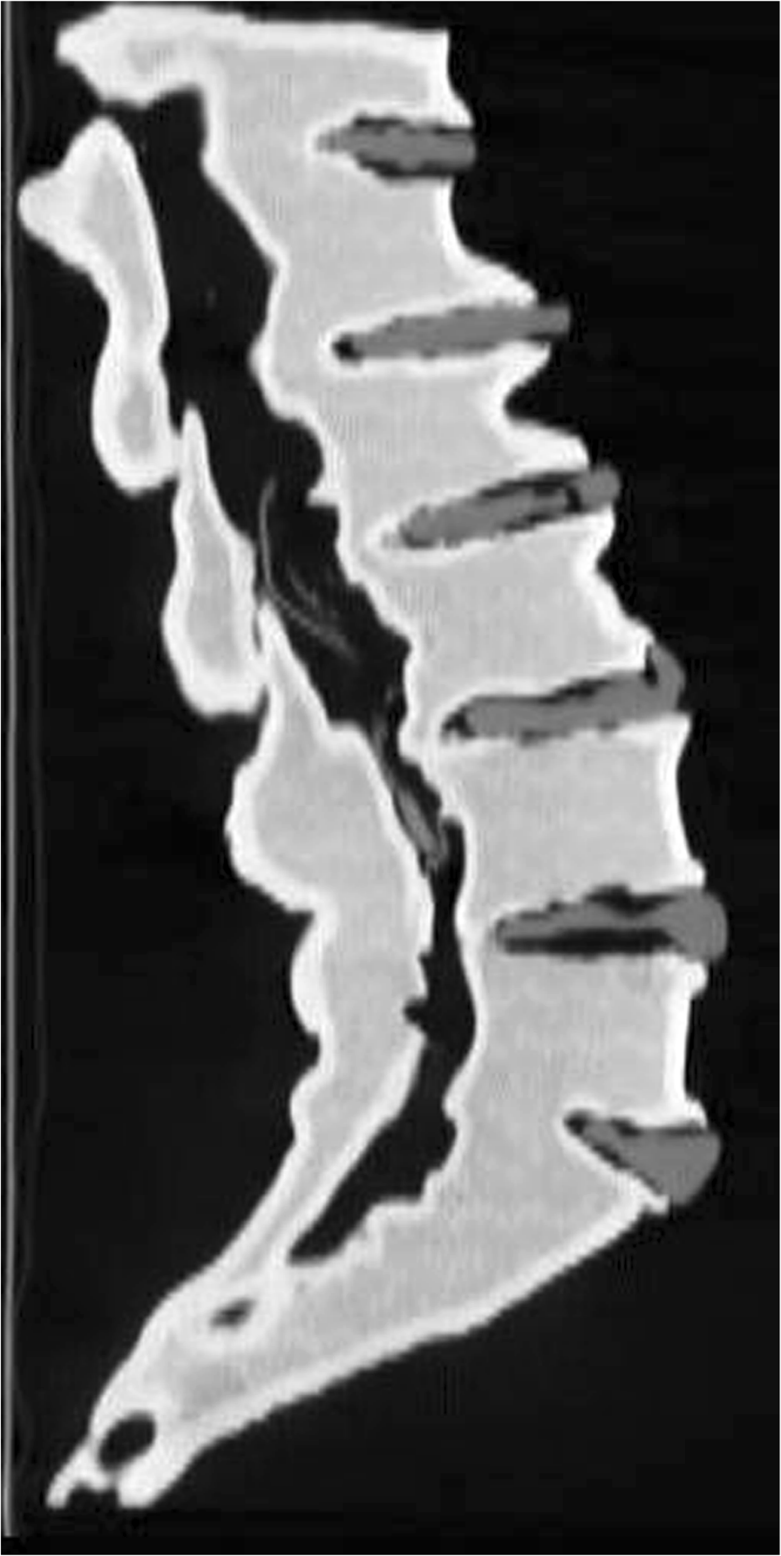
Sagittal reformat of CT of the lumbar spine model, demonstrating differential densities and ability to distinguish the vertebral bodies, discs and nerve roots
